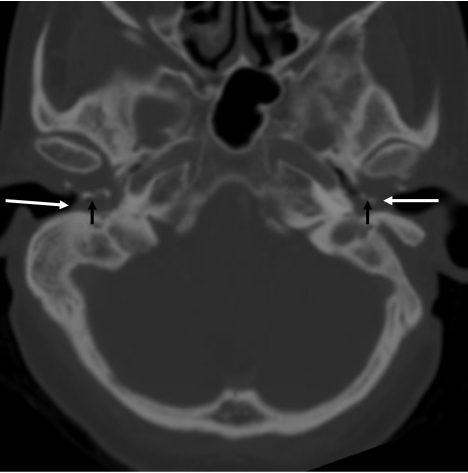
CT of temporal bone - axial image, in bone window showing soft tissue filling up the external auditory canal and the middle ear (long white arrows). The bony fragments are clearly seen in this window. (short black arrows).
